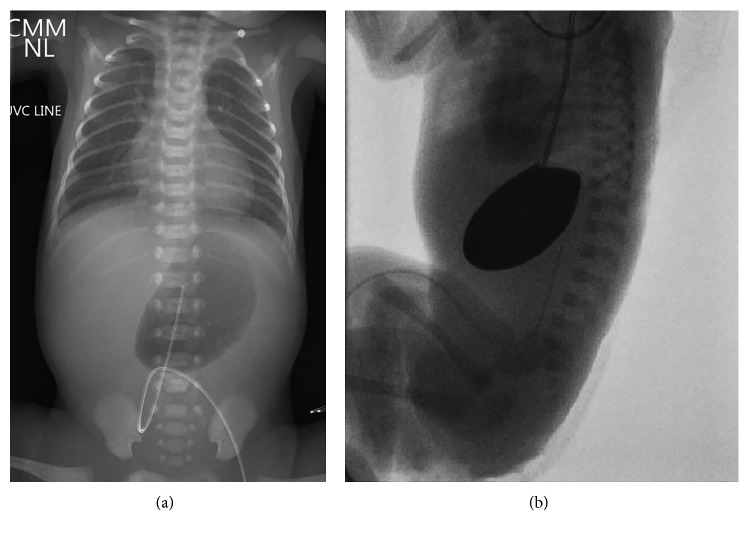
Radiographic imaging consistent with pyloric atresia. (a) Abdominal X-ray showing gaseous distension of the stomach with no free intra-abdominal air. (b) Fluoroscopic abdominal X-ray showing total gastric outlet obstruction with no contrast entering the duodenum.
